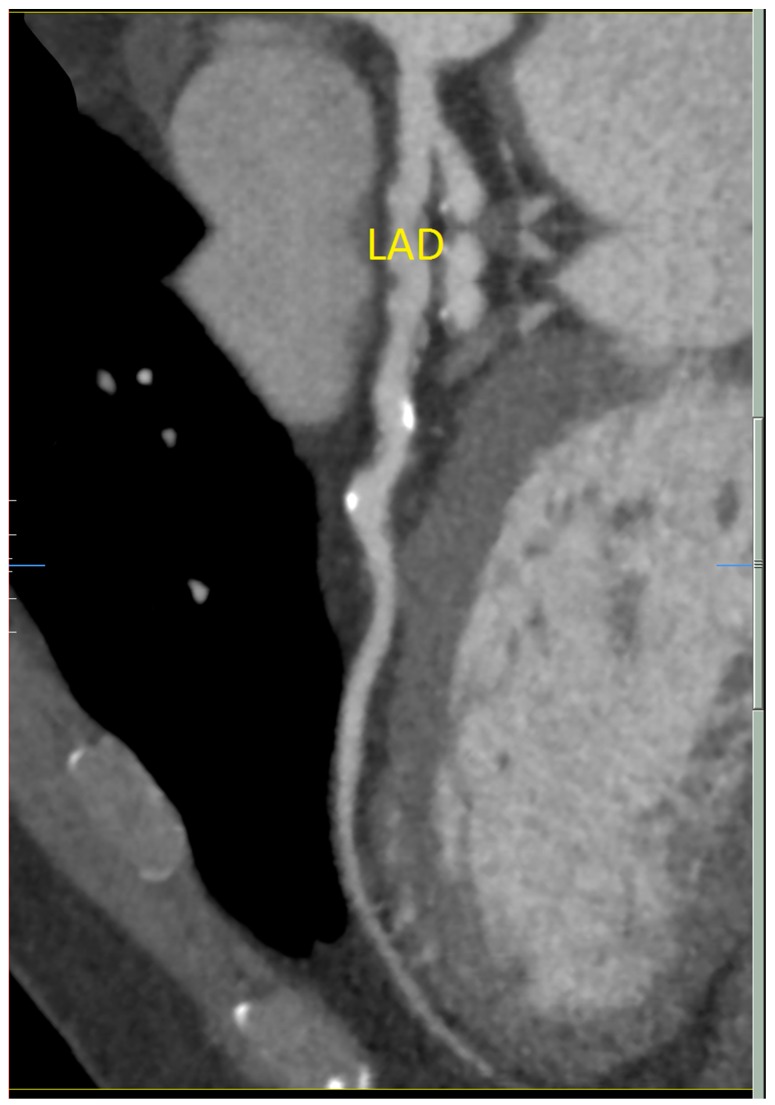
Cardiac computed tomography showing diffuse ectasia of the left anterior descending (LAD) artery.
